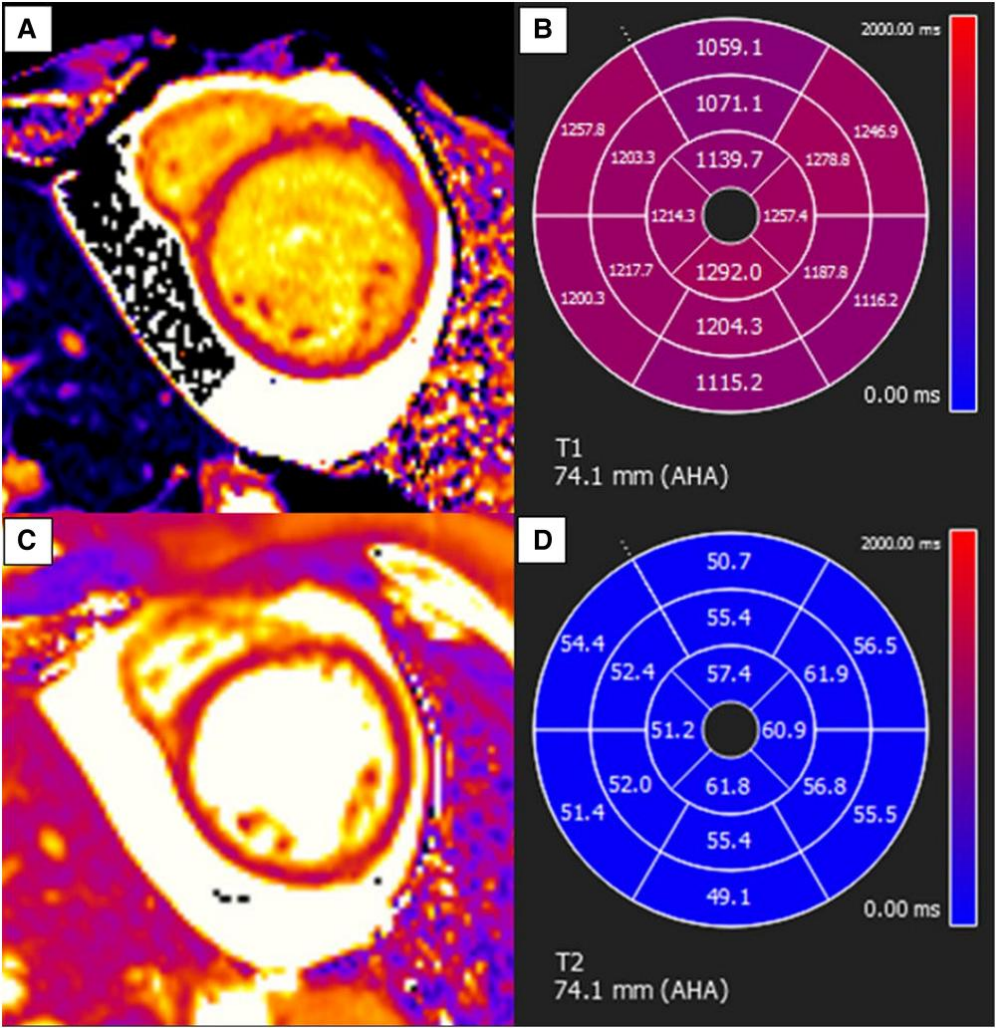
Cardiac magnetic resonance images. (*A*) T_1_ mapping (modified Look–Locker inversion recovery sequences) image at the mid left ventricle. (*B*) T_1_ polar map shows elevated values in all segments in keeping with diffuse interstitial fibrosis. (*C*) T_2_ mapping image at the mid left ventricle. (*D*) T_2_ polar map shows elevated values at all the lateral segments as well as the mid to apical anterior and inferior segments. Native T_1_ mapping normal range: 902–1054 ms; T_2_ mapping normal range: 39–54 ms.
